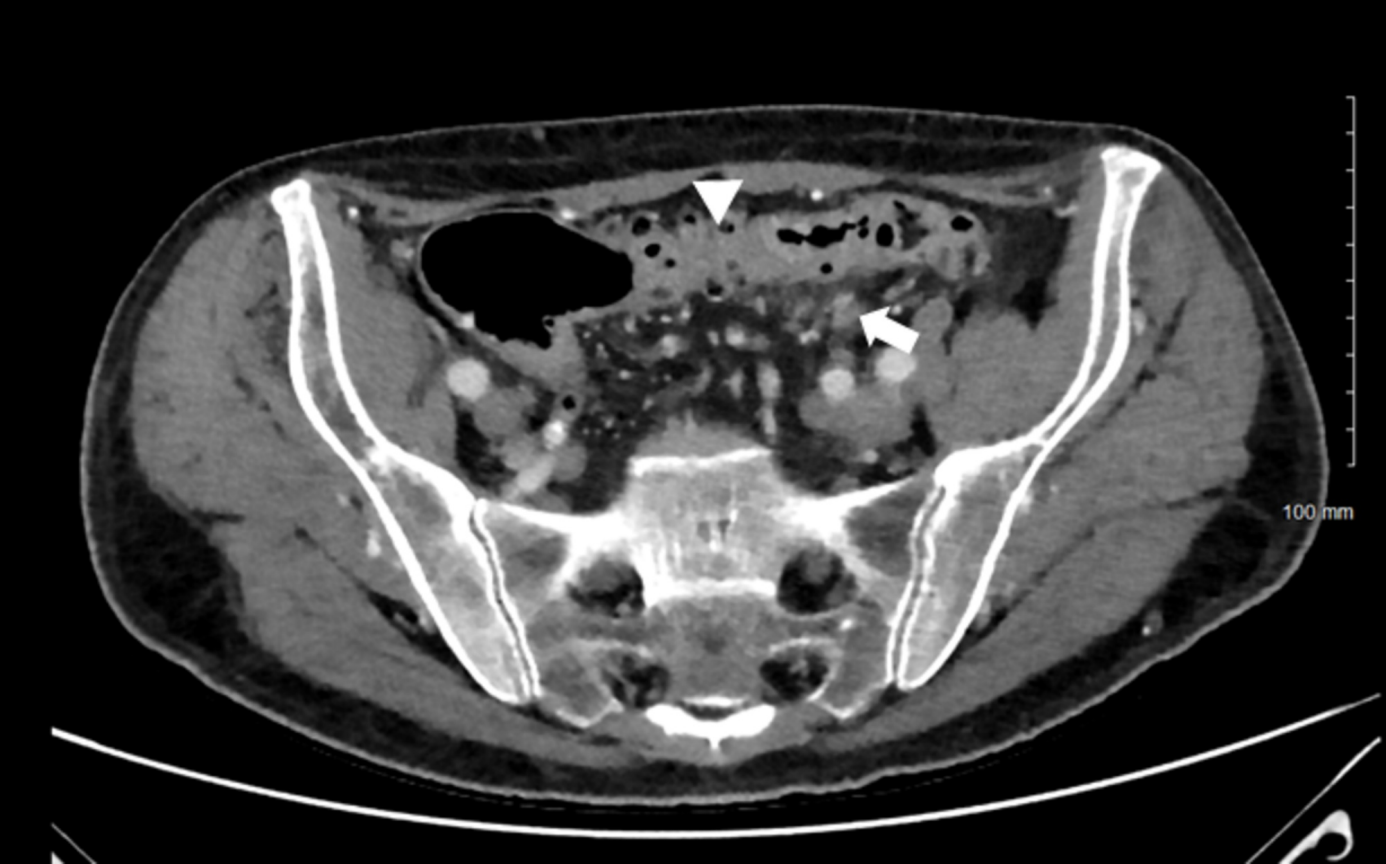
CT of the abdomen obtained after alleviation of symptoms continues to show perisigmoid fat stranding and wall thickening. Wall thickening is marked with an arrow head and the perisigmoid fat stranding is marked with an arrow.
